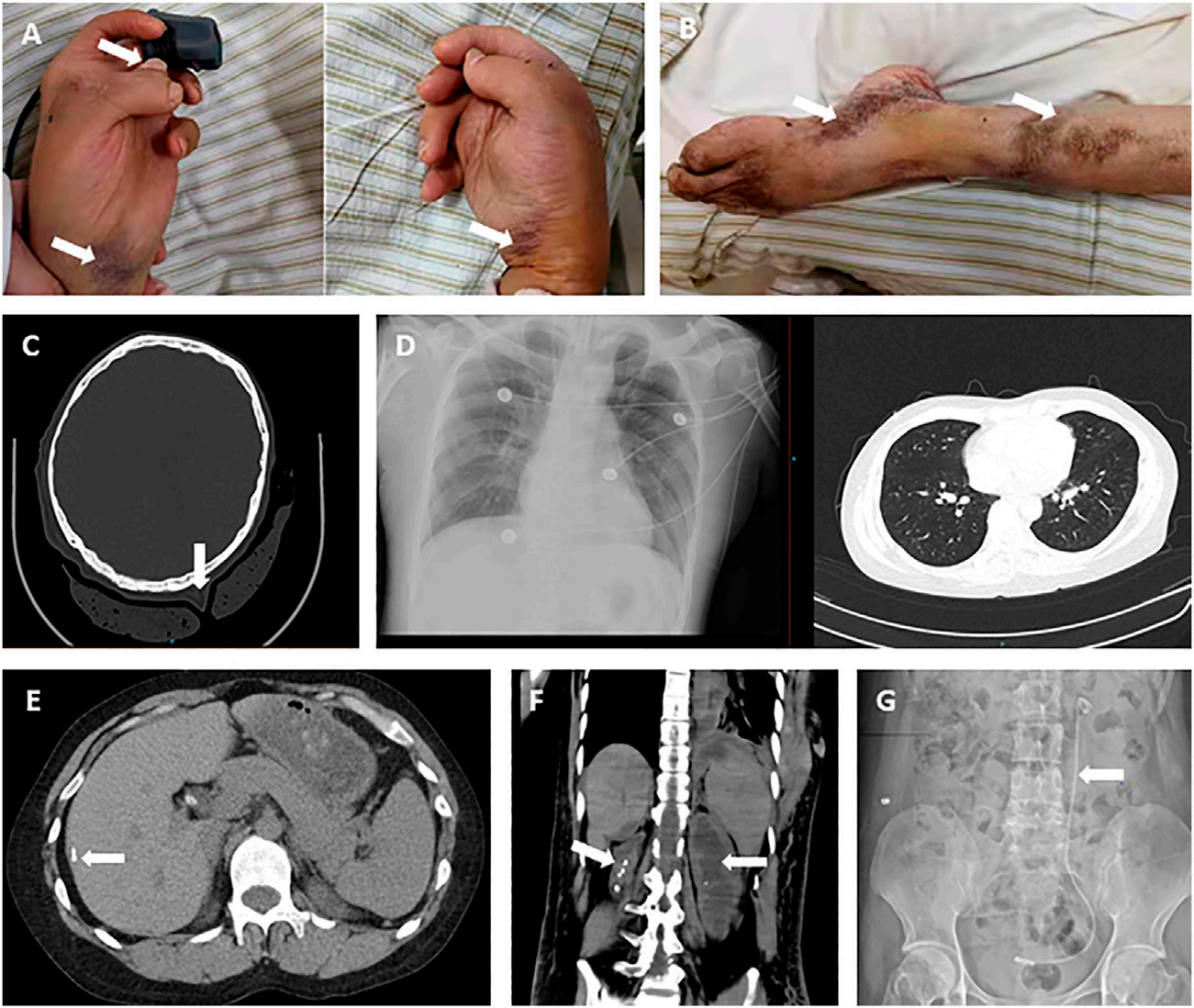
Patient’s clinical images at admission. (A) Claw-shaped hands with multi-finger deformity of the left thumb, with easily bruised and over stretchable skin (arrow). (B) Foot drop deformity (left), with surgical scars on the skin of the leg. (C) Over stretchable soft tissue of head shown on CT image. (D) X-ray images were presented for the lung in normal shape and size. (E) Calcifications in the liver. (F) Kidney atrophy (right), hydronephrosis, and renal calculi. (G) J stent in the urinary tract (left).
